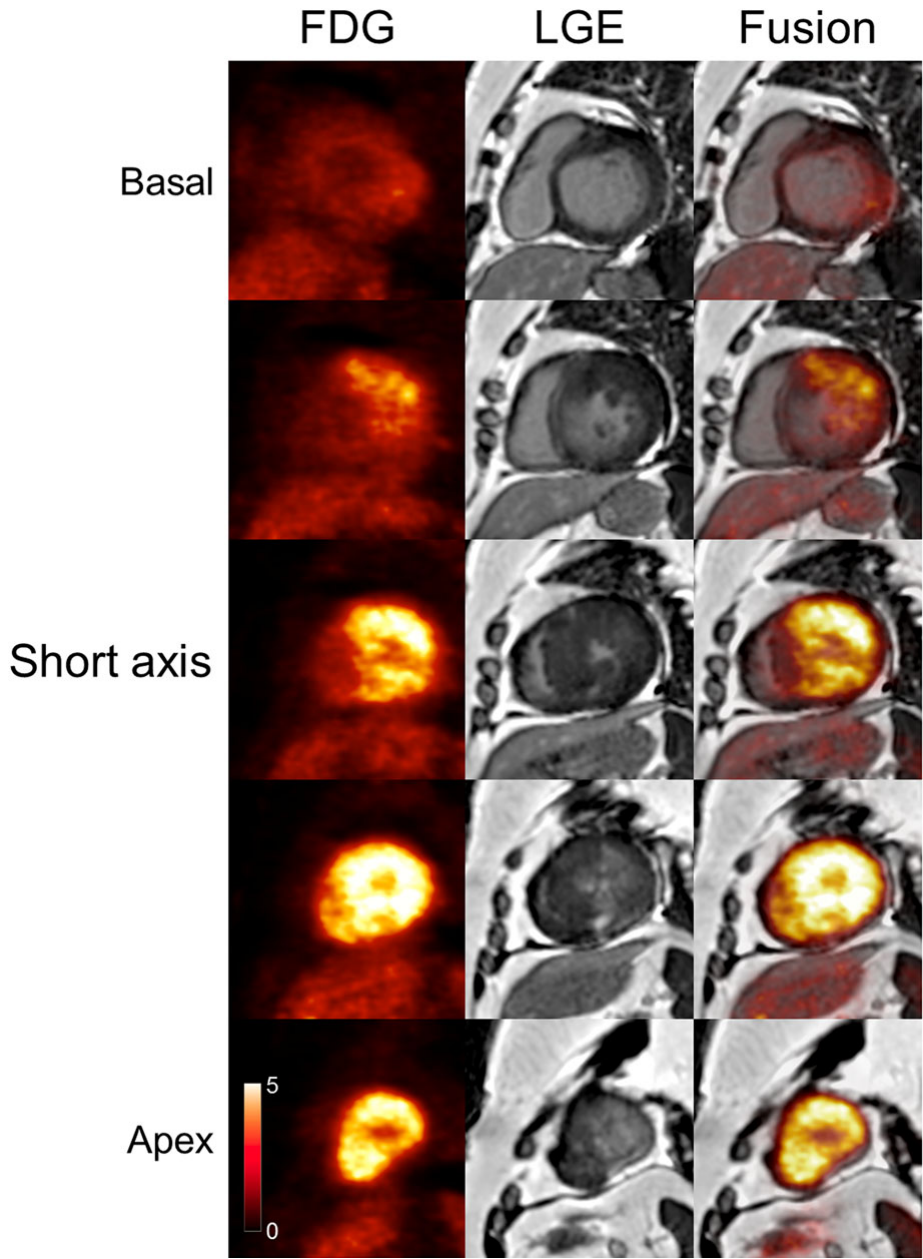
Serial short-axis images of ^18^F-fluorodeoxyglucose PET/MRI. ^18^F-fluorodeoxyglucose (FDG), late gadolinium enhancement (LGE), and fusion images from base (*upper*) to apex (*lower*)
